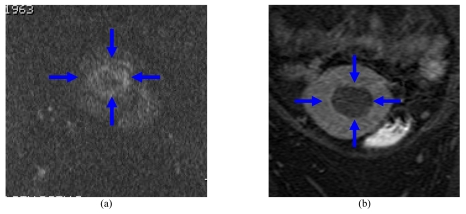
(a) Coronal DWI images compared to (b) post-contrast fat suppressed T1WI to assess the extent of ablation (arrows) at the same level.
